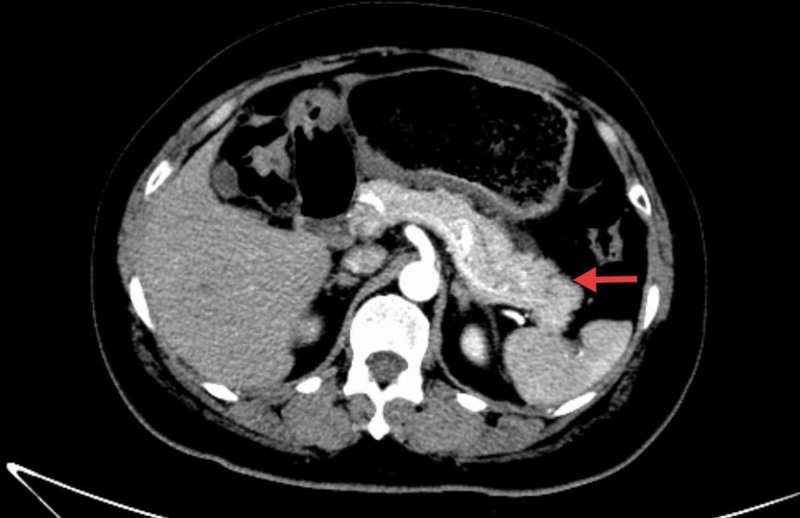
Contrast-enhanced CT of the abdomen. The red arrow indicates mild swelling of the pancreas. CT = computed tomography.
